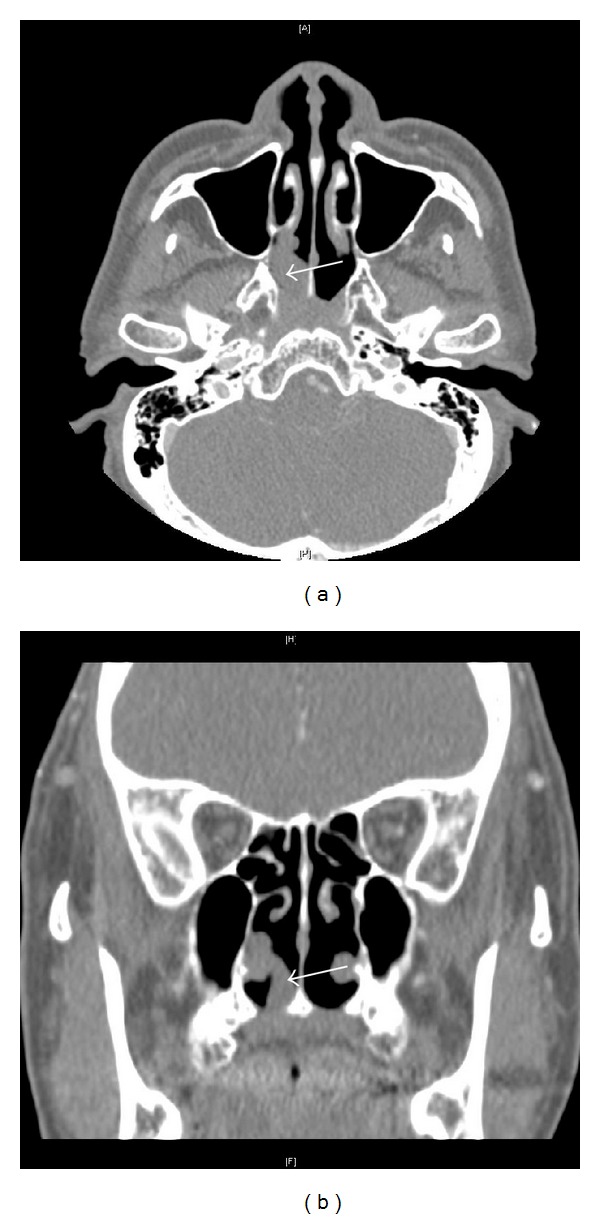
Preoperative paranasal sinus CT. Nasal polyp originating from the posterior portion of the right inferior turbinate is protruding into the choana ((a) axial view, (b) coronal view).
